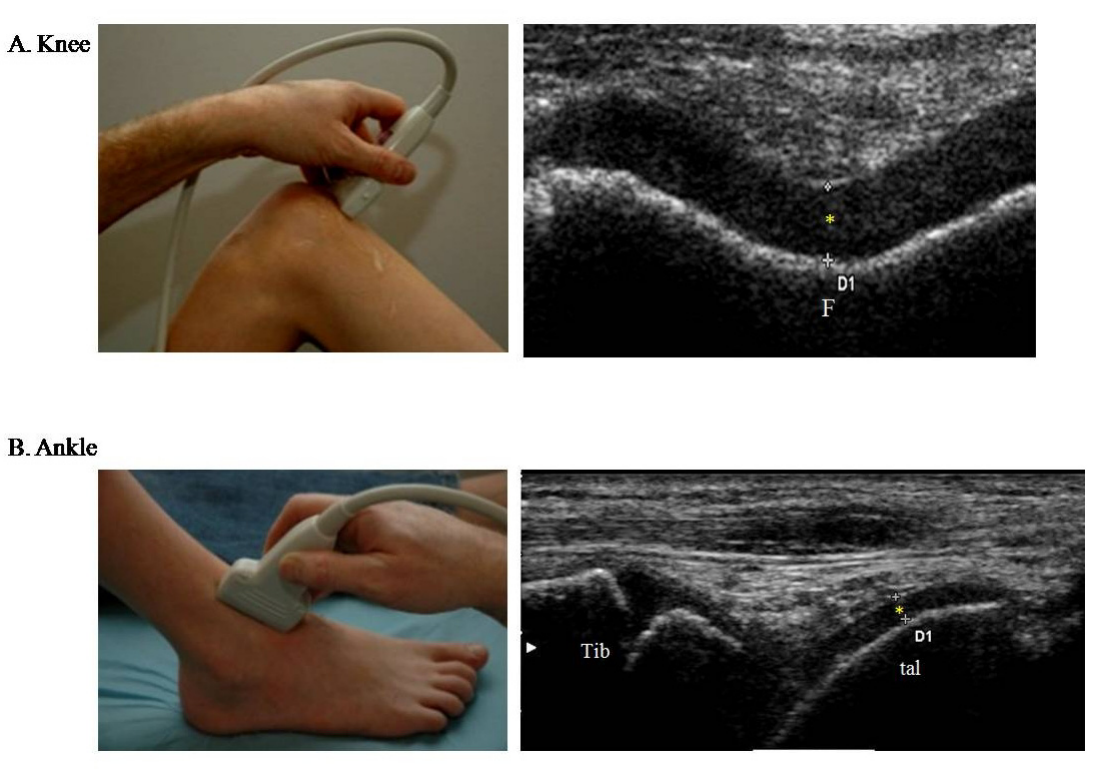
US ultrasonographic standard scans for cartilage thickness measurements in the knee and ankle joints. * = hyaline cartilage, F = femur, Tib = tibia, tal = talus.
